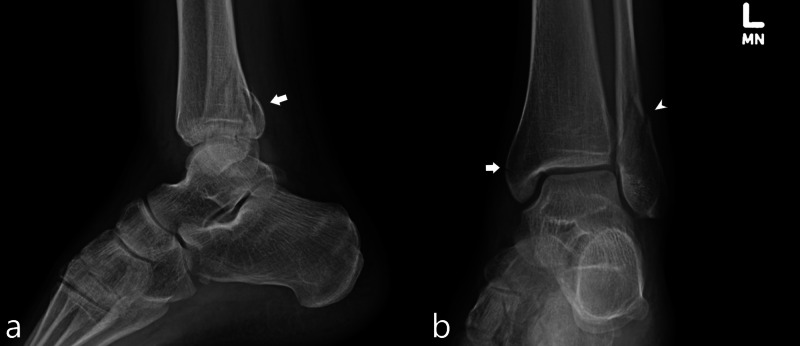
Case 5 ankle radiographs A lateral radiograph of the ankle (a) demonstrates a mildly displaced posterior malleolar fracture (white arrow). An oblique mortise view radiograph of the ankle (b) demonstrates a nondisplaced fracture of the medial malleolus (white arrow) and the lateral malleolus (white arrowhead).
